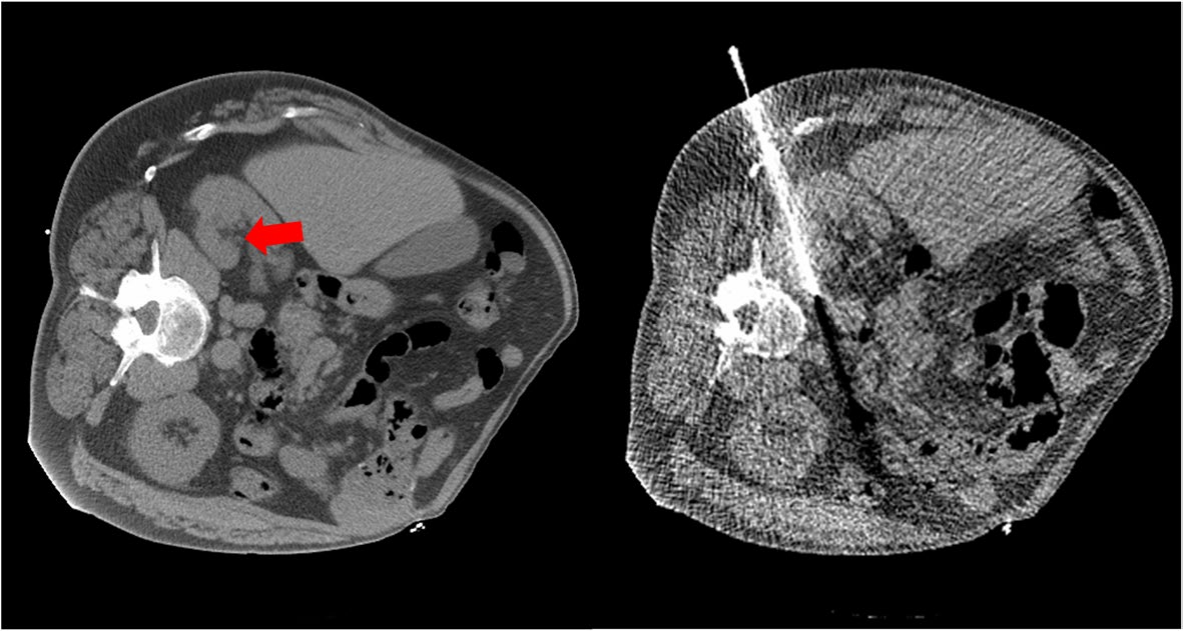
Metal artifacts from biopsy needles can obscure targets. In this example, a planning CT is shown on the left, and an intraprocedural CT fluoroscopy image on the right. The target is a 1.8 cm lymph node which is easily visualized on the left image (red arrow) but completely obscured by an artifact on the right. Interventional device artifacts commonly manifest themselves in this way, hindering both visualization of important anatomy that will be traversed by the needle, and localization of the target
